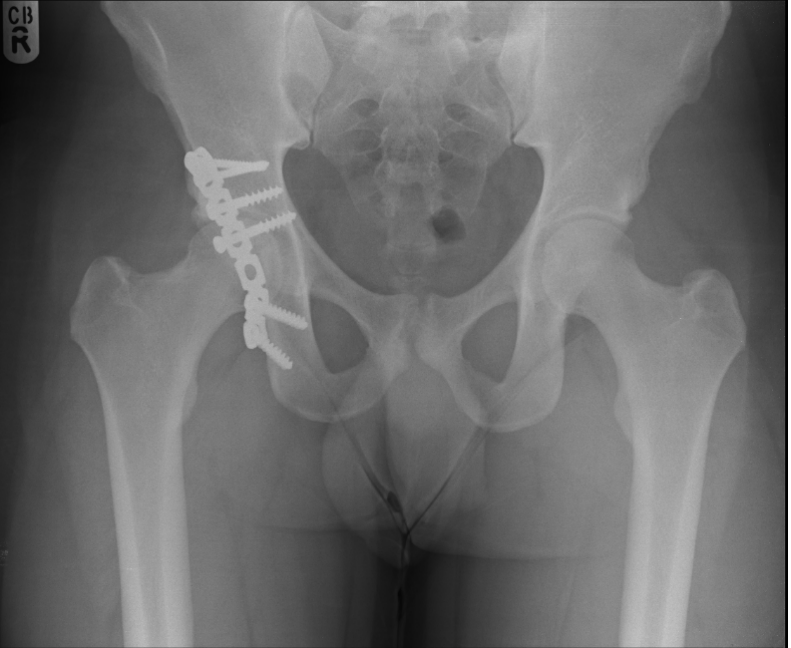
One year follow up radiograph.
